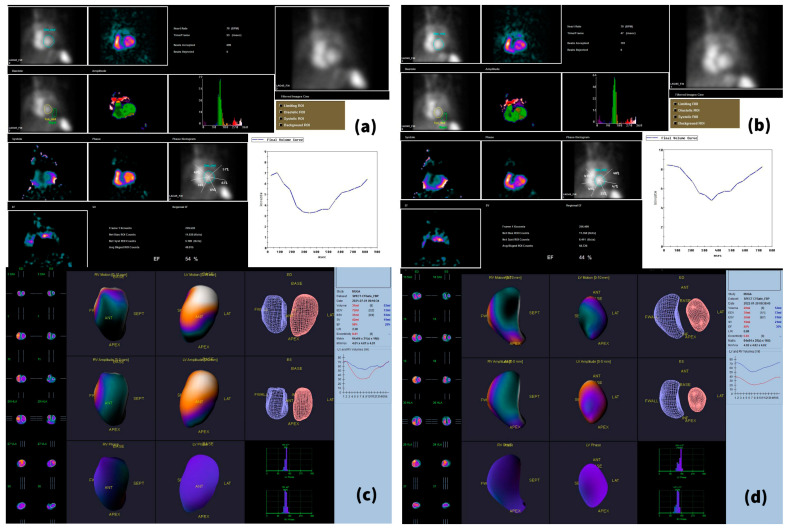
Patient fulfilling the ESC criteria for cardiotoxicity. This 64-year-old female patient suffered from HER2 3+ invasive ductal carcinoma, ER- and PR-negative, previously treated with anthracyclines. During the 6-month period, she received six cycles of trastuzumab, pertuzumab and docetaxel. A decline in LVEF can be observed in both planar and SPECT images, with the EF diminishing from 54% at T0 (a) to 44% at T1 (b) on planar scans, and from 58% (T0—(c)) to 48% (T1—(d)) on the SPECT acquisitions.
